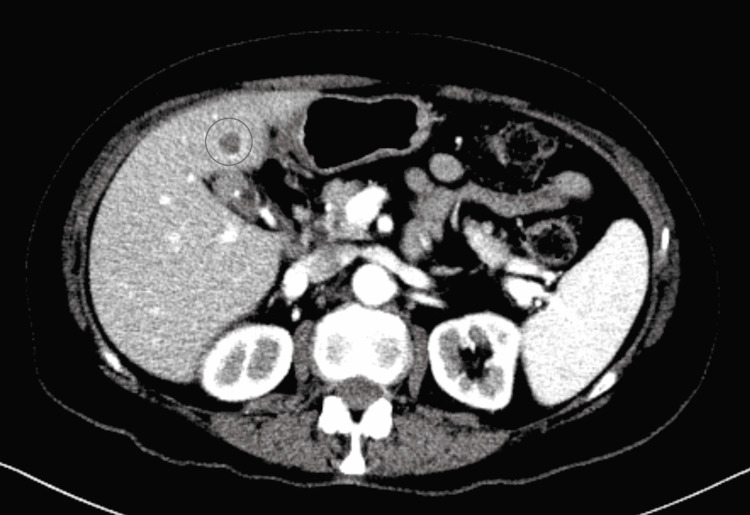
CT of the abdomen and pelvis with contrast showing 1.1 cm hypodense lesion within segment IVb This CT image is used here in the article with written consent from the patient's legal guardian.
